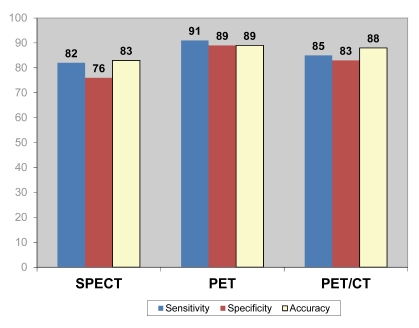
Overall pooled mean diagnostic value of SPECT, PET and PET/CT for detection of coronary artery disease.
